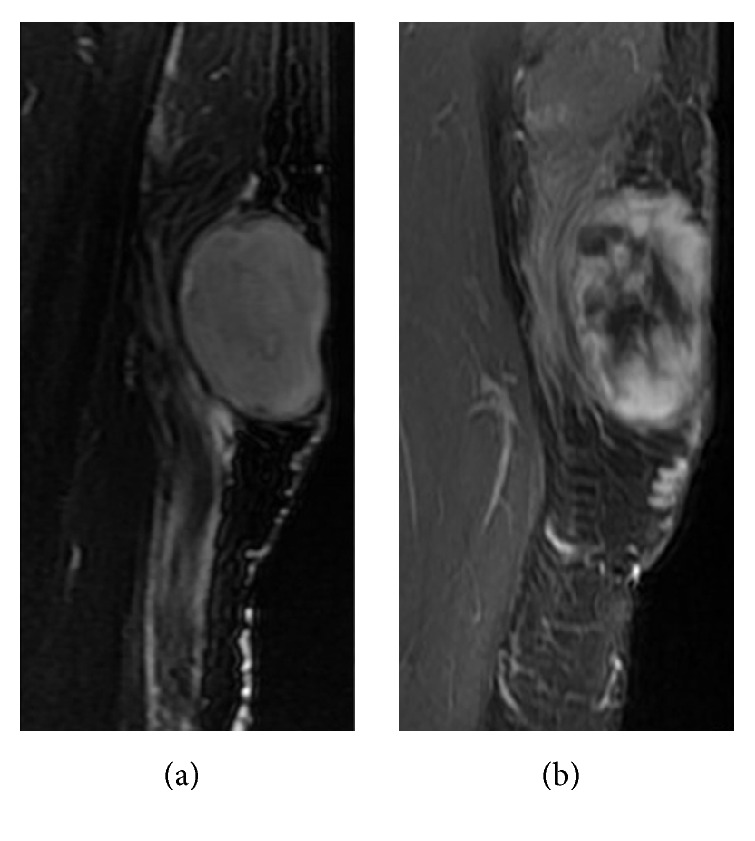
Images obtained from a 44-year-old female with IMT of the right thigh. (a) An oval-shaped soft tissue mass in the right thigh with long T2 was observed. (b) An enhanced MRI scan revealed heterogeneous enhancement.
